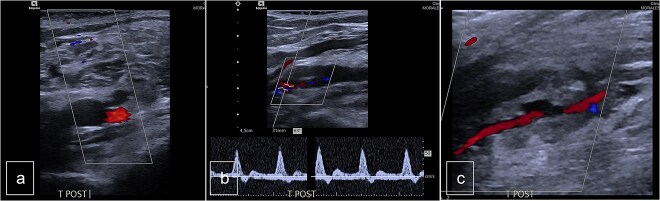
Postoperative duplex sonography. (a) Doppler mode. Identification of the PTA. (b) Doppler mode. Triphasic physiological flow in distality of the autologous patch. (c) Doppler mode. Permeability of the PTA.
